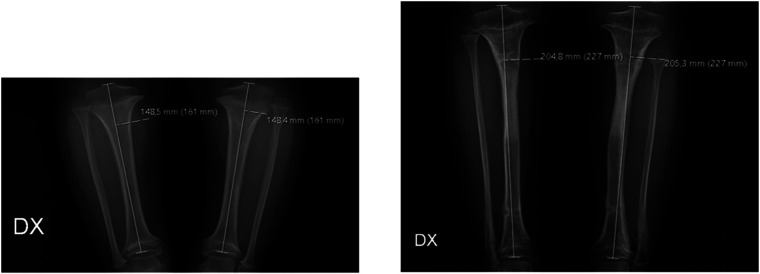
Radiographic measurements of the tibia before surgery (left image) and after removal of the external fixators (right image).
